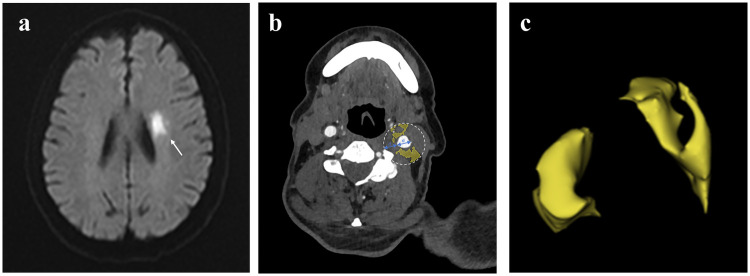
Segmentation of PVAT on CTA. (a) A case that experienced a stroke; (b) PVAT (yellow) segmented automatically by the segmentation software; and (c) an image showing the three-dimensional PVAT region (yellow).
